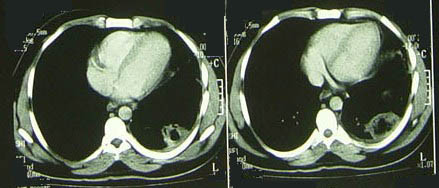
CT scan showing left lower lobe consolidation with cavitation and moderate pleural effusion.
